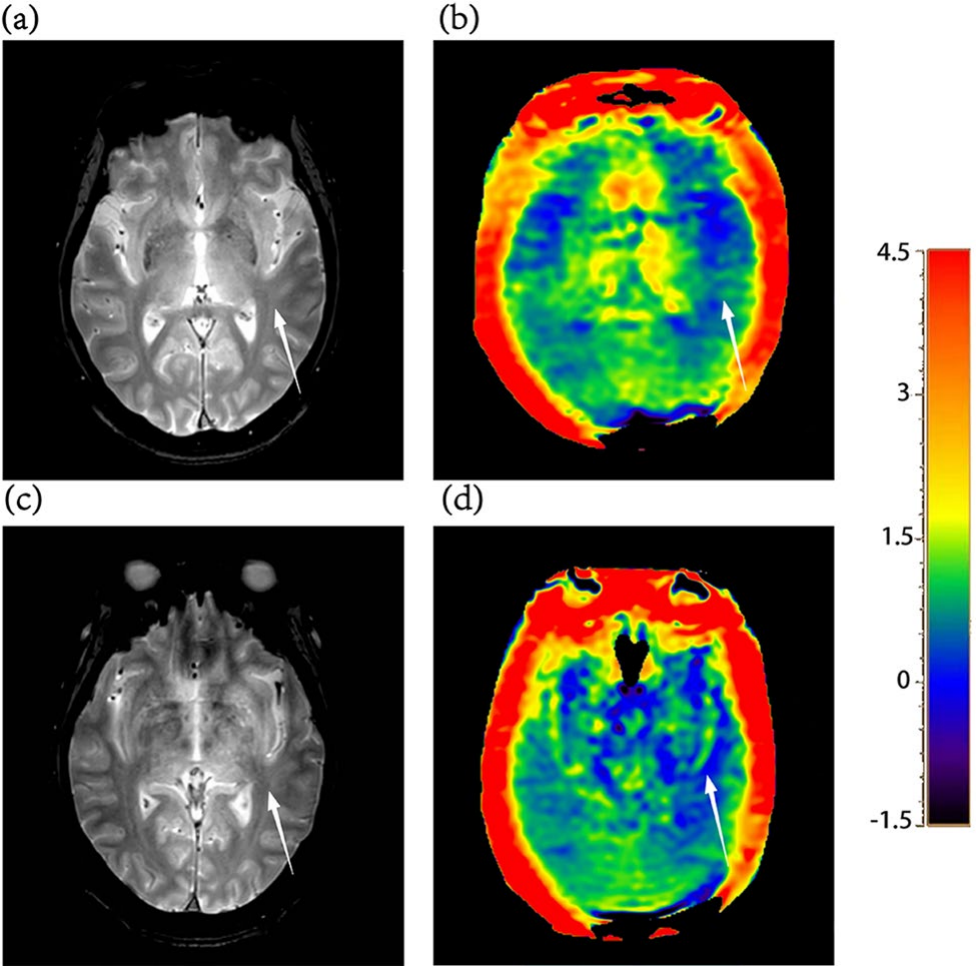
a T2-weighted image and b APT-weighted image of a healthy control (male, 61 years old, MoCA score 26). c T2-weighted image and (d) APT-weighted image of a T2DM-MCI patient (female, 68 years old, MoCA score 23). The APT-weighted signal (white arrow) in the left TWM region was lower in the T2DM-MCI patient than in the healthy control
